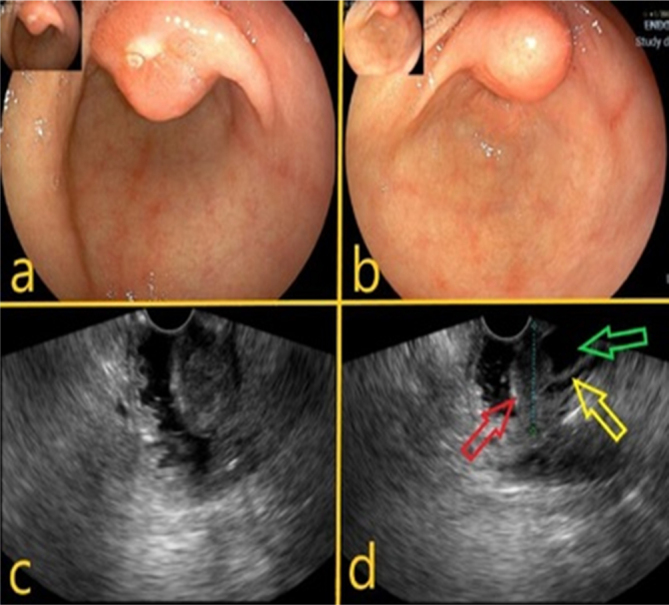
Endoscopic and endoscopic ultrasound images of major duodenal
